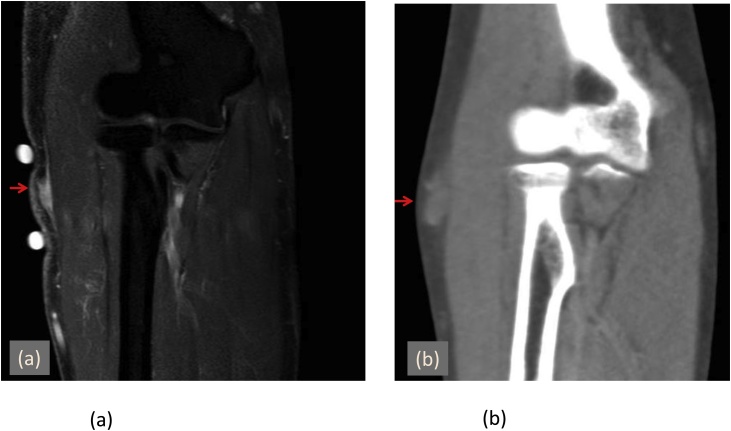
Comparison of the initial and recurrent tumors. (a) MRI showed a mass (9 × 6 mm) that was thought to be nodular fasciitis. (b) Four years later, the recurrent subcutaneous mass (23 × 10 mm) was difficult to diagnose by CT.
